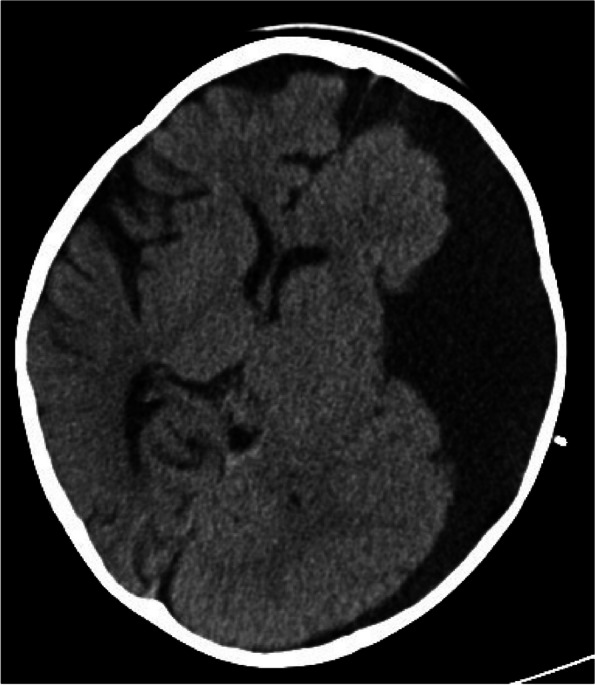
Follow-up cranial CT scan 4 days later showing increased subdural effusion with a more pronounced midline shift
